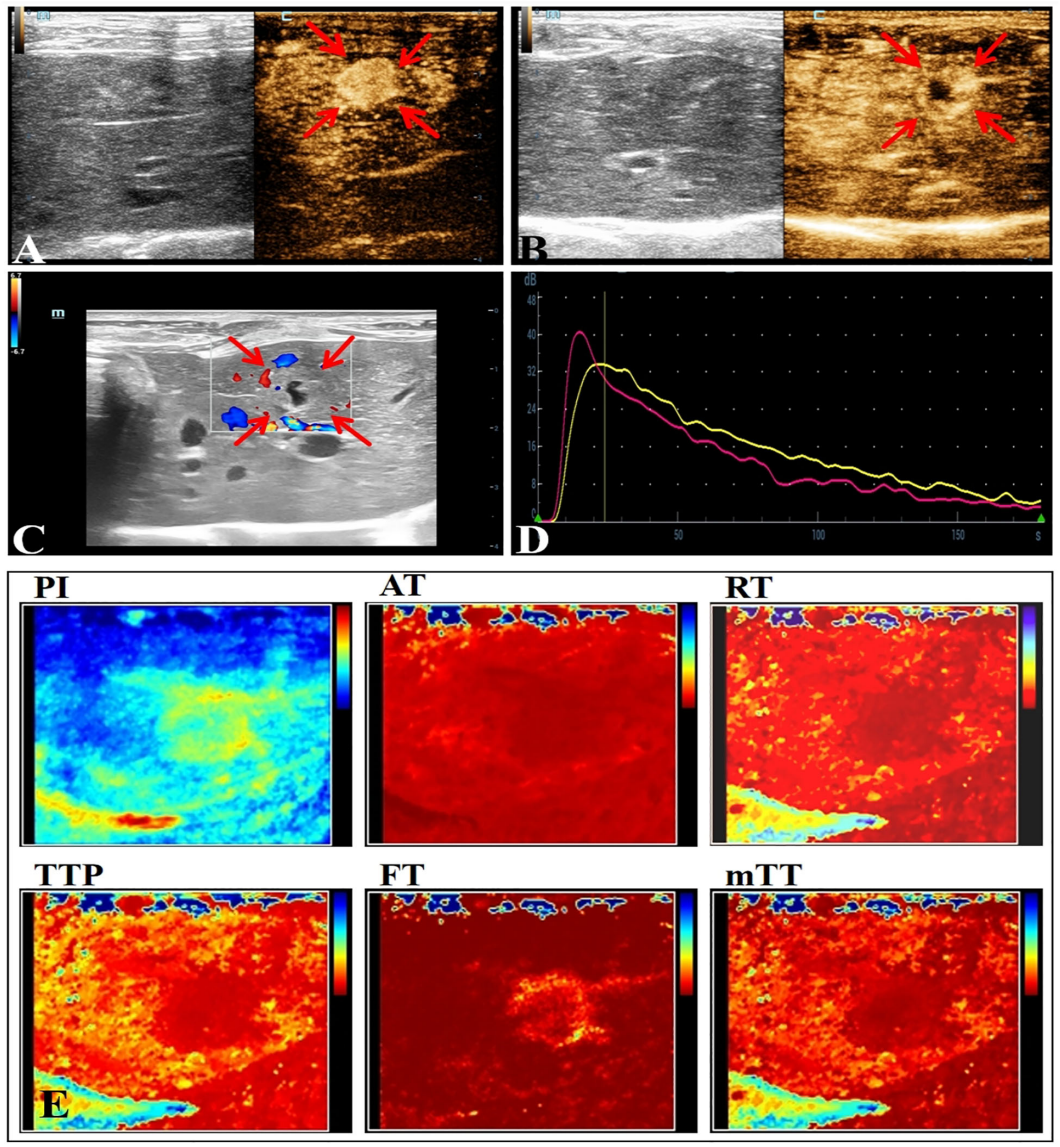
Conventional ultrasound and CEUS manifestations of VX2 tumors in rabbit liver. (A) The arterial phase of CEUS shows overall hyperenhancement. (B) The arterial phase of CEUS shows peripheral hyperenhancement. (C) Ultrasonographic presentation of rabbit liver VX2 tumor with nodular echoes close to the liver, unclear borders, punctate blood flow signals seen within, and irregular necrotic cystic areas seen in the center. (D) CEUS TIC curve. (E) Visualization image of the CEUS TIC curve parameters of VX2 tumor.
